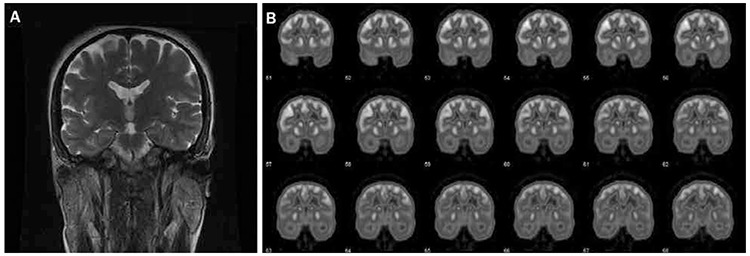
Coronal magnetic resonance imaging (MRI) view (A) and interictal fluorine-18 fluorodeoxyglucose (F-18 FDG) positron emission tomography (PET) study (B) of a 27 year old male patient with intractable complex partial seizures. The electroencephalogram recordings of the patient displayed bilateral temporal lobe abnormalities, while the MRI was normal (nonlesional temporal lobe epilepsy). Interictal F-18 FDG PET study showed hypometabolism on the right temporal lobe. At the postoperative (right anterior temporal lobectomy) follow up, the patient was seizure free (Engel I outcome)
